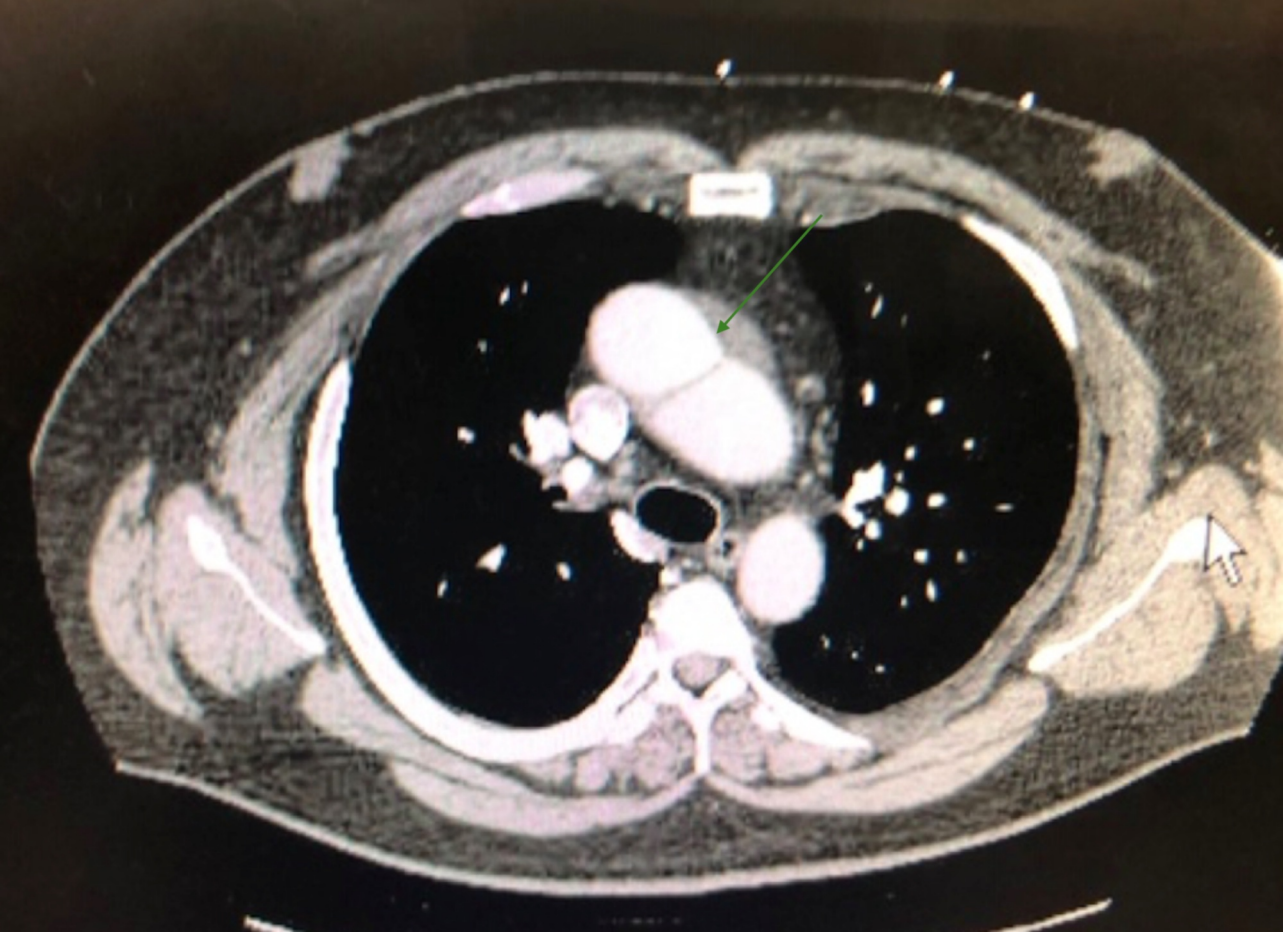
Ascending aorta dissection CT scan of the chest with intravenous contrast showing the aortic dissection.
